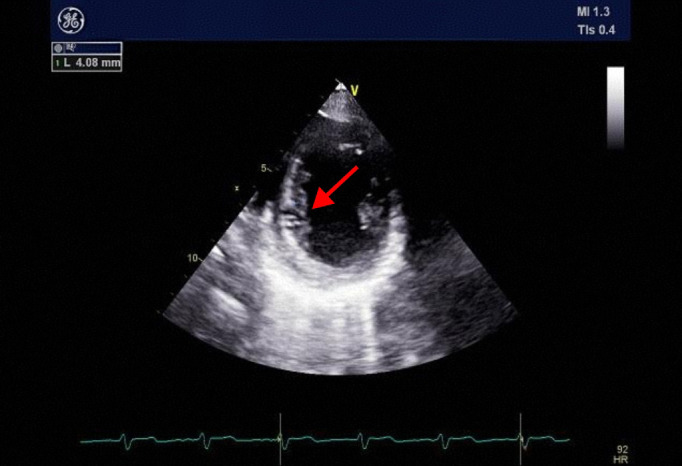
Transthoracic echocardiography on admission. The study reveals significant thickening of the left ventricular endocardium (arrow, max 4.08 mm), a characteristic finding suggestive of eosinophilic infiltration.
